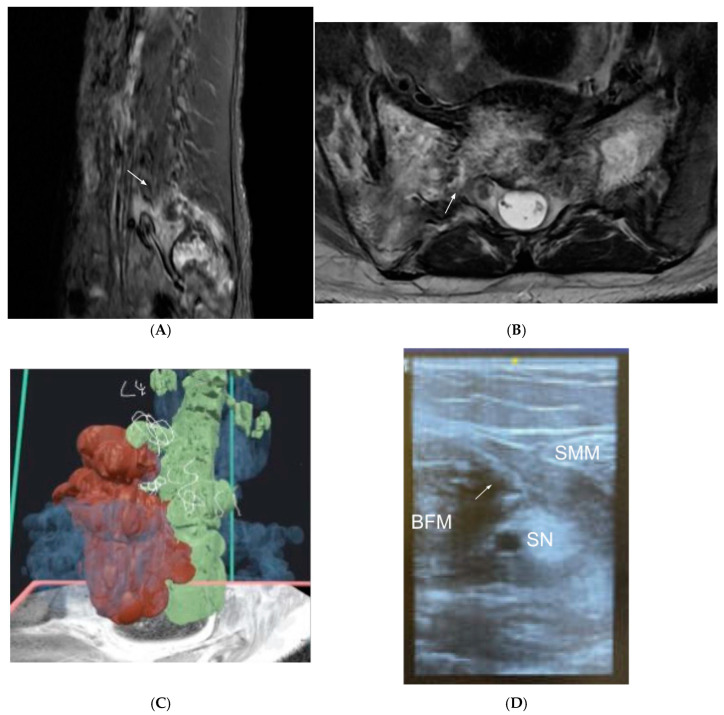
Patient 4, who developed significant radicular pain in the sciatic nerve distribution from a large, destructive lymphoma, causing spinal and foraminal stenosis. (A,B) Initial imaging prior to chemotherapy showing a sacral mass with epidural extension and significant right > left L5/S1 foraminal stenosis. White arrows indicate the mass. (C) Manual segmentation was utilized to generate a 3D model of the patient’s spine MR. Grayscale was manually converted to color by our team, with blue representing bone, green representing neural structures, and red demarcating the tumor. (D) Ultrasound-guided sciatic PNS lead deployment near the popliteal fossa. BFM = biceps femoris muscle, SN = sciatic nerve, SMM = semimembranosus muscle, white arrow indicates PNS lead.
